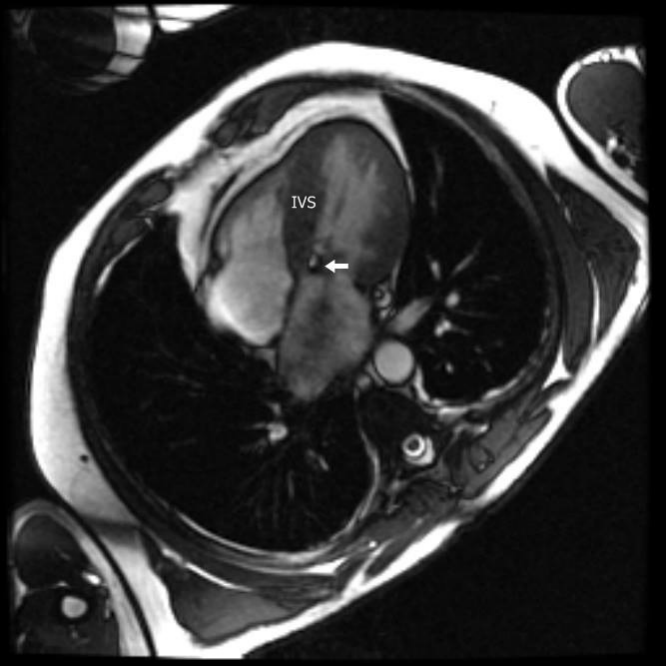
Four-chamber cardiac magnetic resonance image demonstrates marked hypertrophy of the IVS in a patient with HCM The arrow indicates the SAM of the MV.
